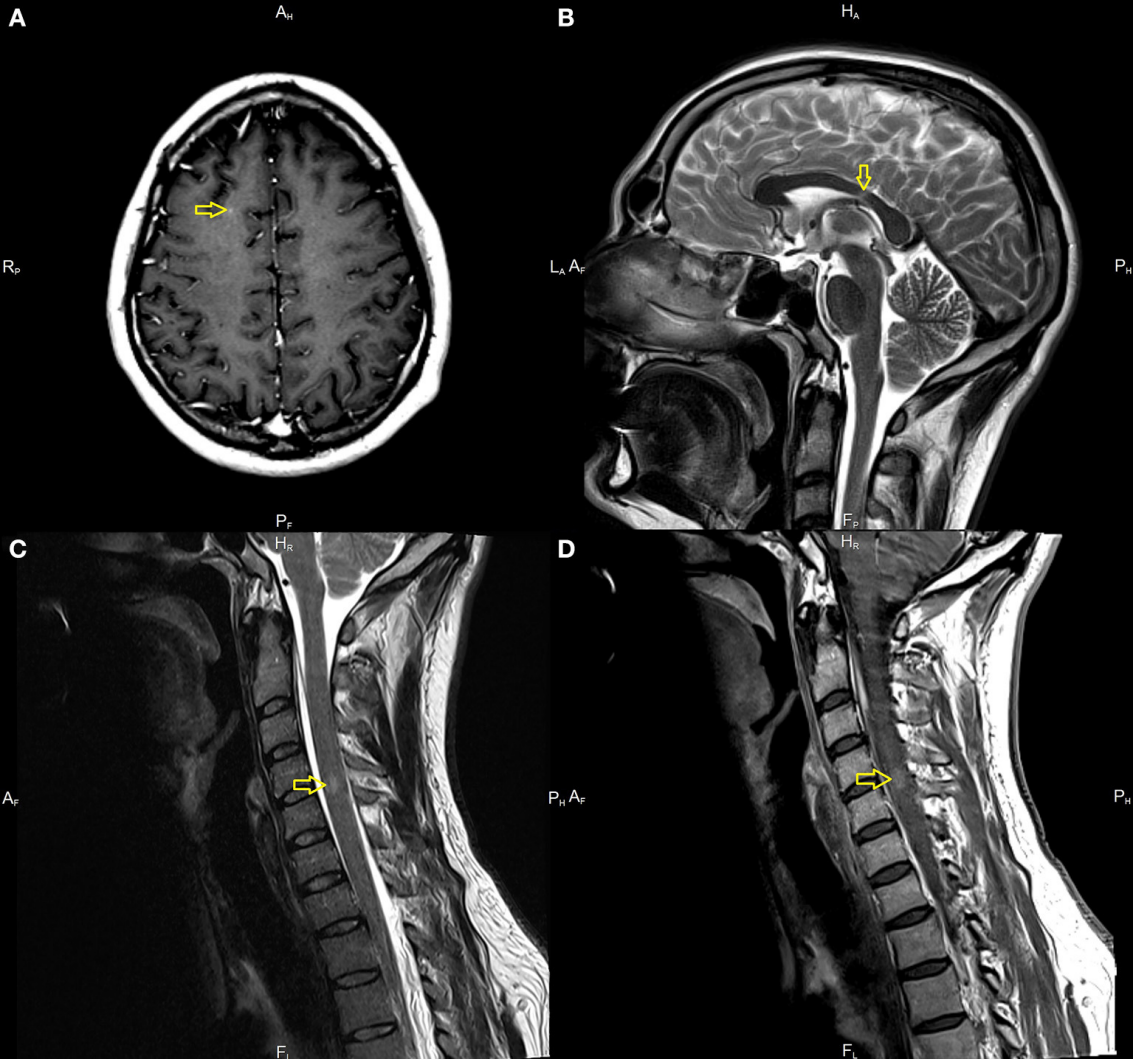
Magnetic resonance imaging (MRI) in the described case of multiple sclerosis (MS). Demyelination lesions are marked with yellow arrows, respectively. (A) T1-weighted subcortical Lesion with gadolinium enhancement on brain MRI on axial sequences. (B) T2-weighted images showing lesion located in corpus callosum. (C) T2 hyperintense acute lesion on cervical spinal cord on sagittal sequences. (D) Gadolinium enhancement of a lesion located in the cervical spinal cord.
